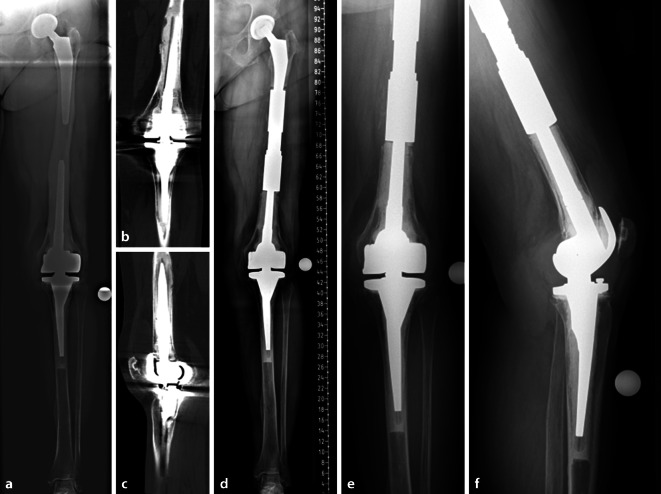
Fallbeispiel: 81 Jahre, weiblich. a Röntgen Ganzbeinstandaufnahme. Festsitzende teilzementierte Hüfttotalendoprothese sowie einliegende femoral und tibial schaftverankerte, zementierte gekoppelte Kniegelenkendoprothese. Der femorale Abstand zwischen den Prothesenspitzen beträgt weniger als 11 cm. Der Isthmus des Markraumes ist eher weit und die kortikale Begrenzung tendenziell ausgedünnt. Um die femorale Schaftspitze der einliegenden Knieprothese zeigt sich ein kortikales „Bowing“ im Sinne einer Stressreaktion. b, c Drei Jahre nach Implantation zeigt sich – in Korrelation zur klinischen Symptomatik – in der CT am ehesten eine interprothetische Insuffizienzfraktur. d–f Postoperative Röntgenkontrolle nach Revisionsoperation mittels Rescue-Sleeve (Fa. Link) unter Erhalt der einliegenden Hüft- und Kniegelenkendoprothese
